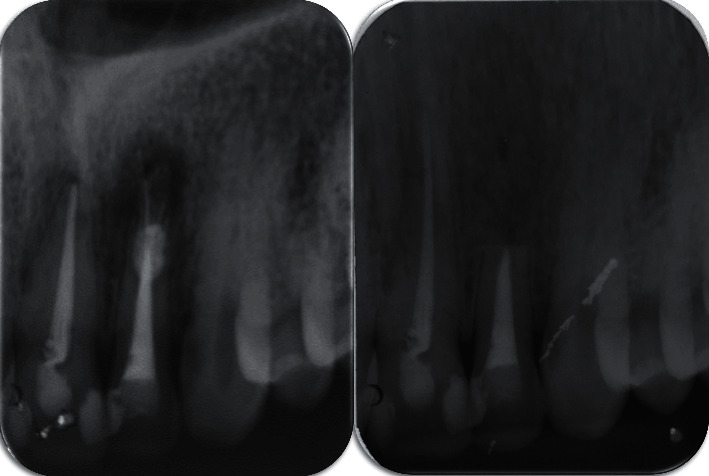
Postoperative periapical radiography immediately after suturing, compared with the initial one.
